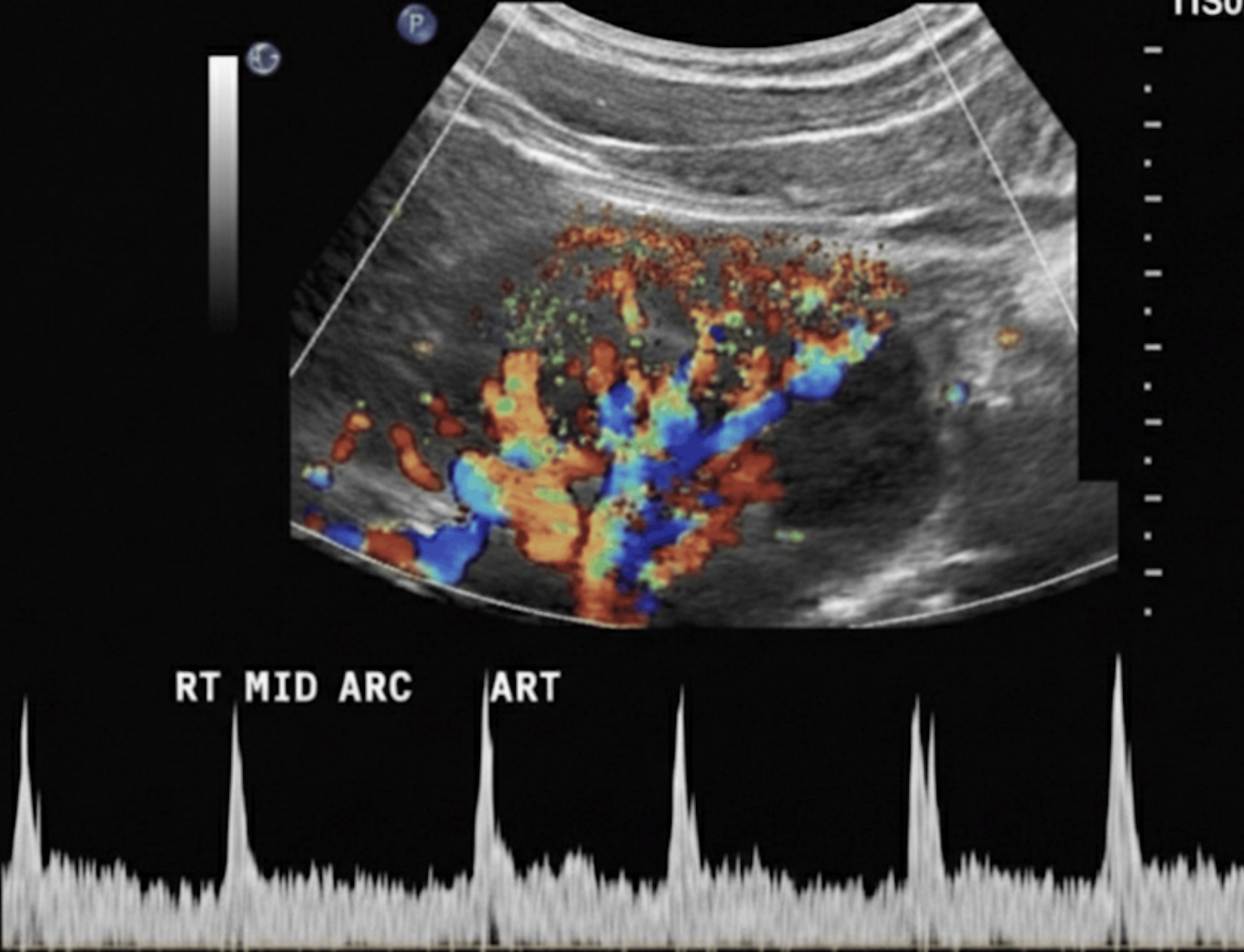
Preoperative Color and Spectral Doppler Ultrasound of the Right Kidney
